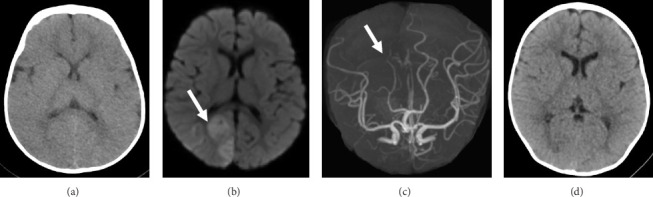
(a): An initial computed tomography (CT) scan image obtained on admission showing no obvious sign of infarction or hemorrhage. (b): A diffusion-weighted magnetic resonance (MR) image on admission demonstrating an acute infarction in the right occipital lobe (arrow). (c): MR angiogram revealing a right parieto-occipital artery occlusion (arrow). (d): A CT scan image on the day after rt-PA administration showing no obvious signs of hemorrhage or infarction.
